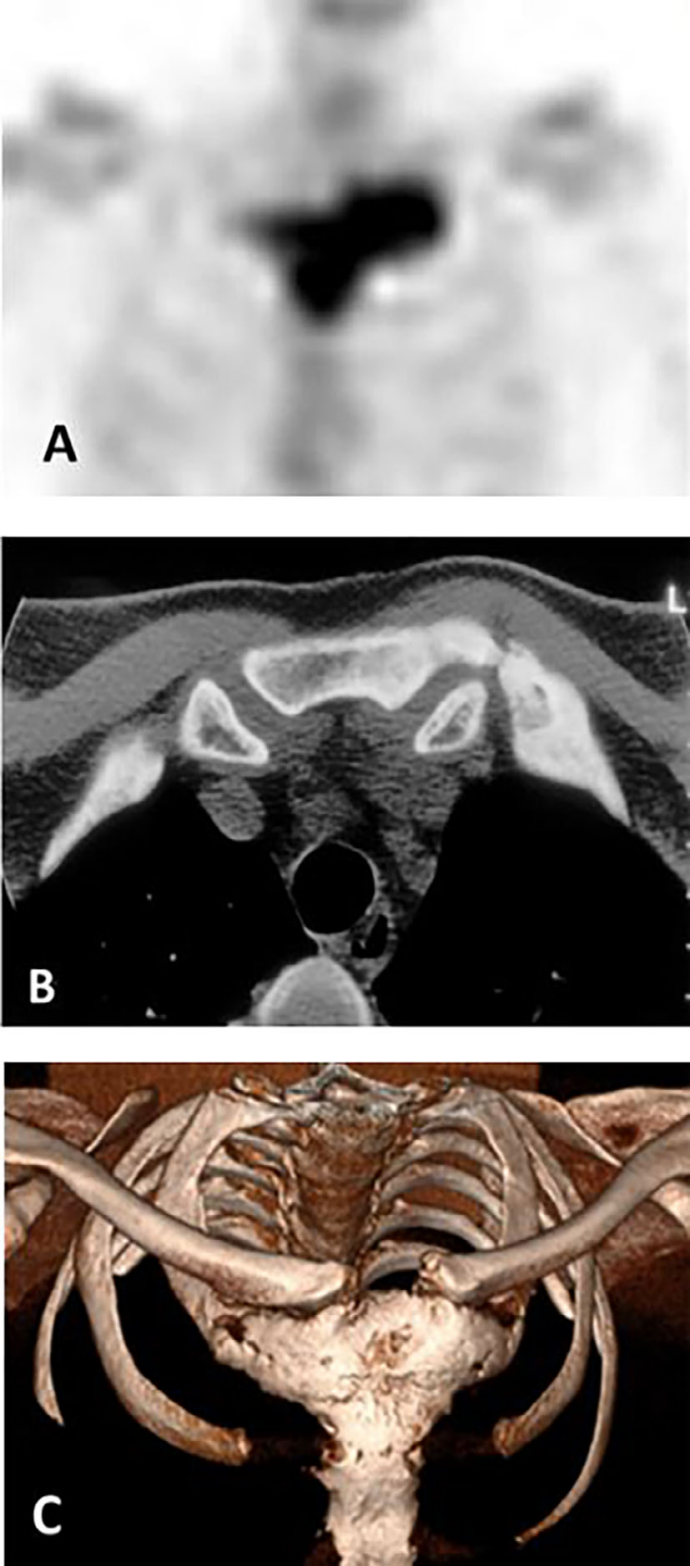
Distinctive imaging features of chronic nonbacterial osteomyelitis (CNO) of the sternocostoclavicular region in an adult patient with CNO/sternocostoclavicular hyperostosis (SCCH). (*A*) ^99m^Tc skeletal scintigraphy showing increased radioactive isotope uptake of the medial end of the clavicle, manubrium sternum, and first rib. (*B*) Distinctive radiological features of sclerosis and hyperostosis of affected bones on transverse CT imaging of the region in the same patient. (*C*) Three‐dimensional image reconstruction of a CT scan of the sternocostoclavicular region in a different patient with CNO/SCCH showing hyperostosis of the manubrium, medial end of the first rib on the left, and of the whole first rib and medial end of the clavicle on the right, forming a large bony costomanubrial bridge and ankylosis of the manubriosternal joint.
